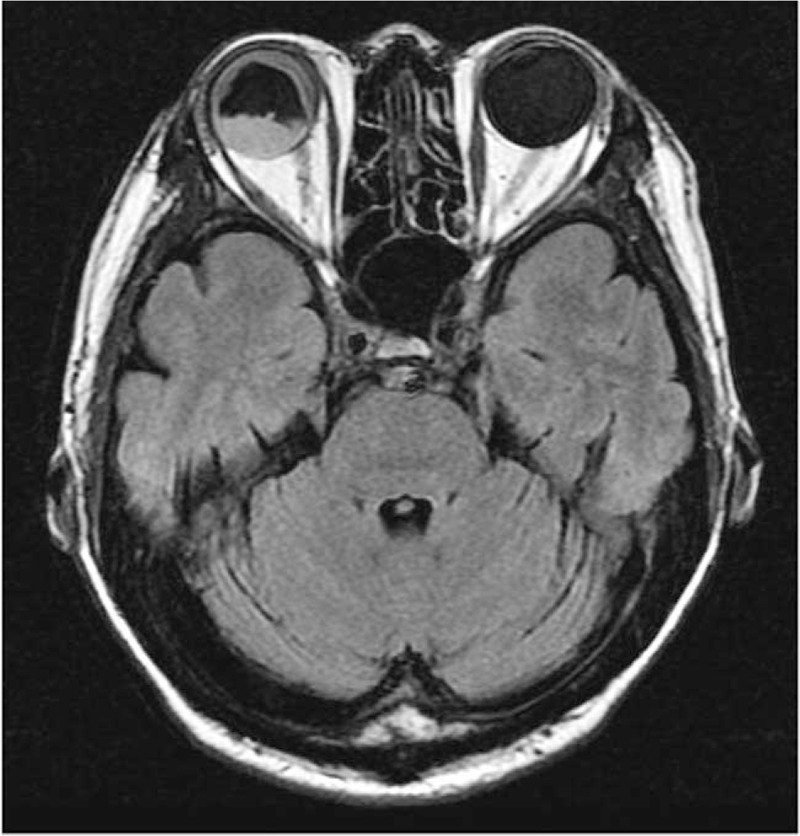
The brain magnetic resonance imaging (MRI) showed a massive high signal lesion in the right eye.
